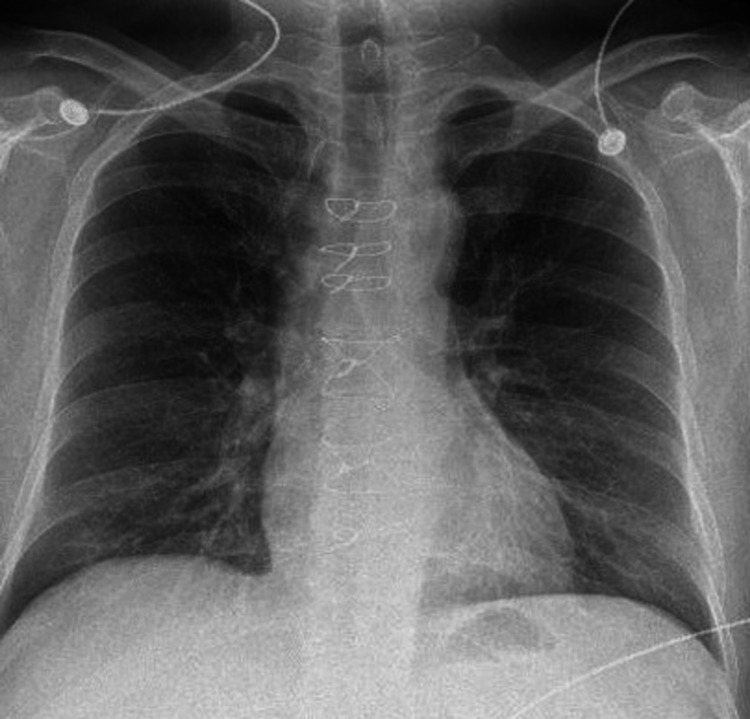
Posteroanterior (PA) chest radiography demonstrating an increasing nodular infiltrate at the left lung apex and post-operative changes in addition to chronic fibrotic.
